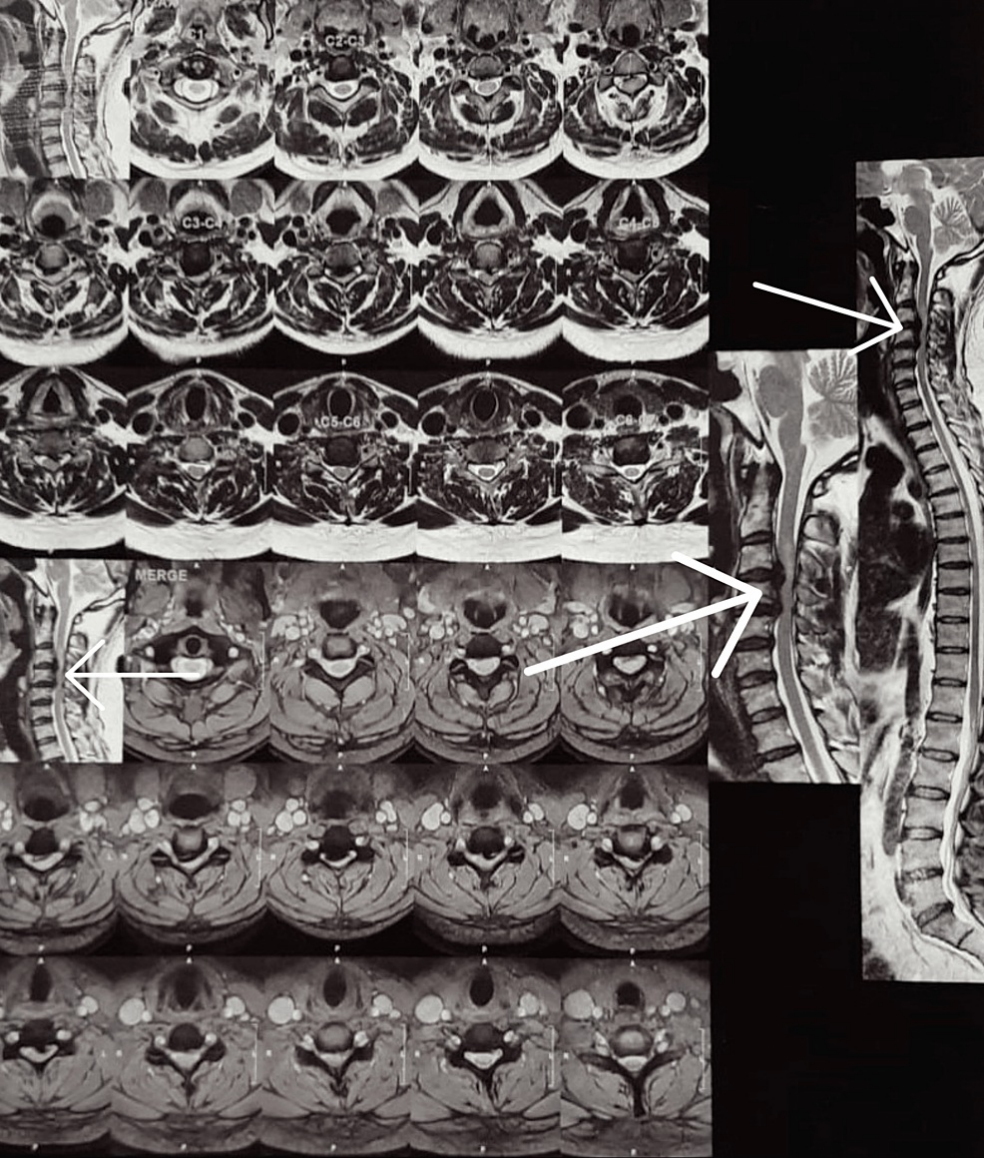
MRI image showing disc bulge Arrows showing areas of disc bulge
